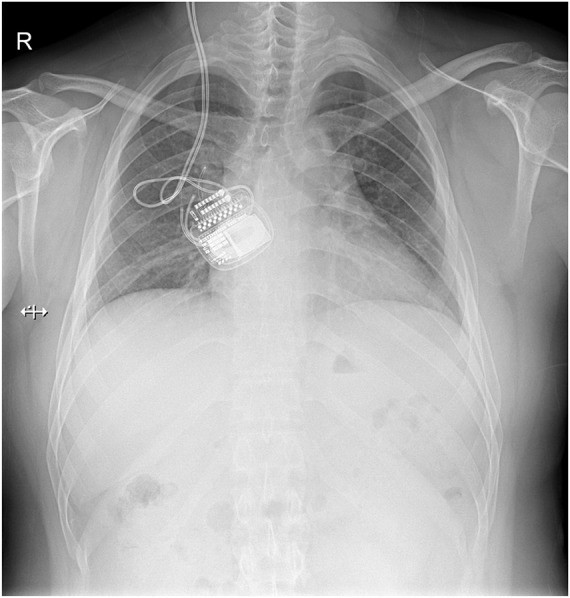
Plain chest X-ray showing the normal view of the implanted pulse generator (IPG) and the extension wire. No fracturing or twisting of the wire was observed and the IPG was in the correct orientation.
